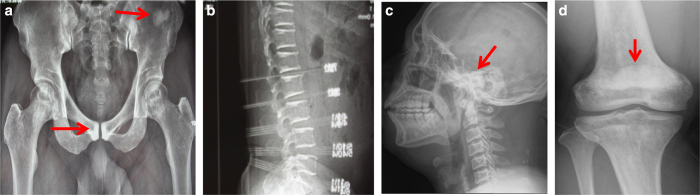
Radiographic examination reveals diffuse osteosclerosis in an ADO2 patient. (a) Bone-in-bone in the pelvis wing (arrow). High-bone density under the cartilage in the pubic symphysis (arrow). (b) ADO2-characteristic sandwich shape of the vertebral body. (c) Osteosclerosis in the base of the skull (arrow). (d) Osteosclerosis in the distal metaphysis of the femur (arrow).
